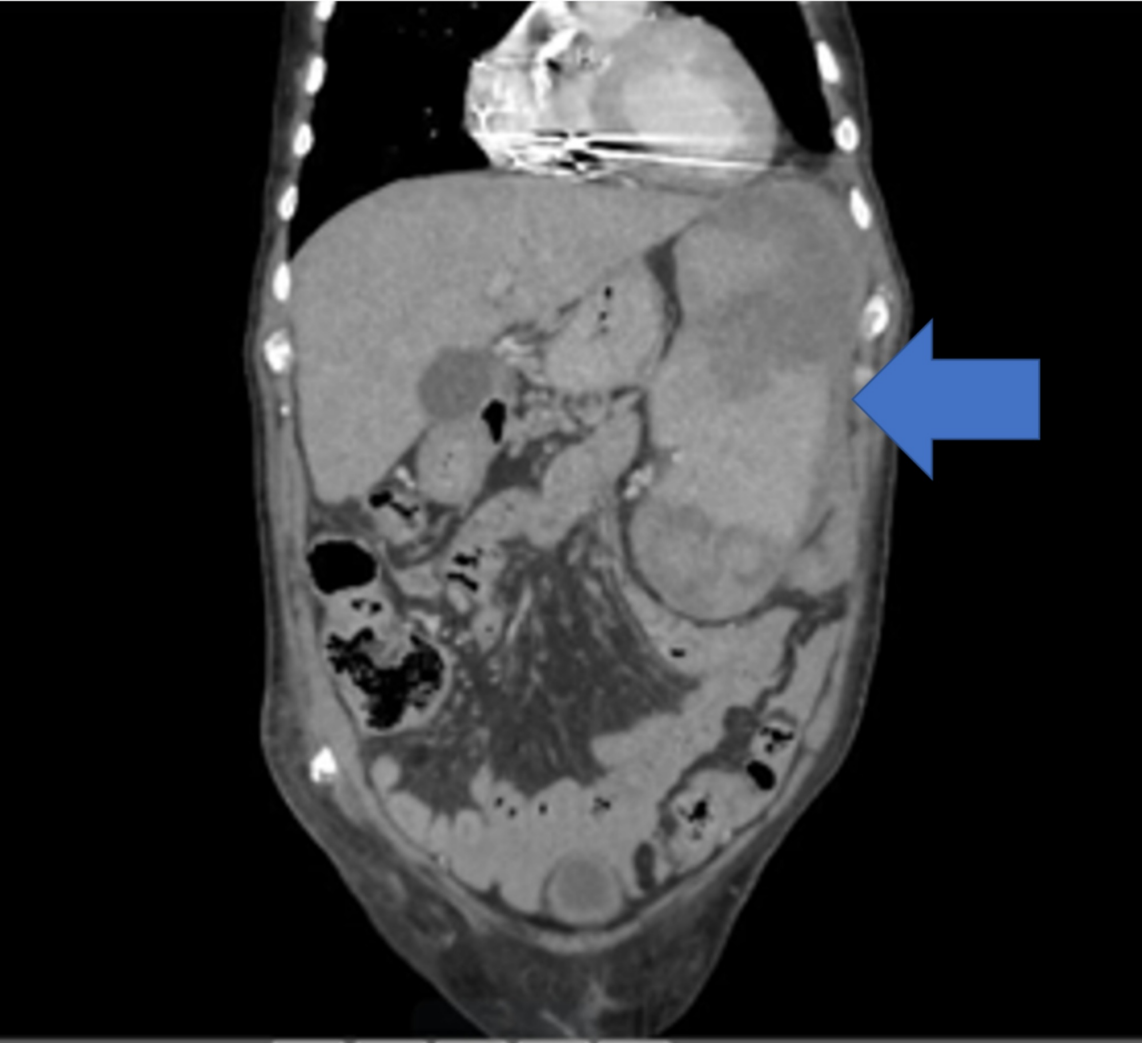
Computed tomography scan showing splenomegaly and splenic laceration
